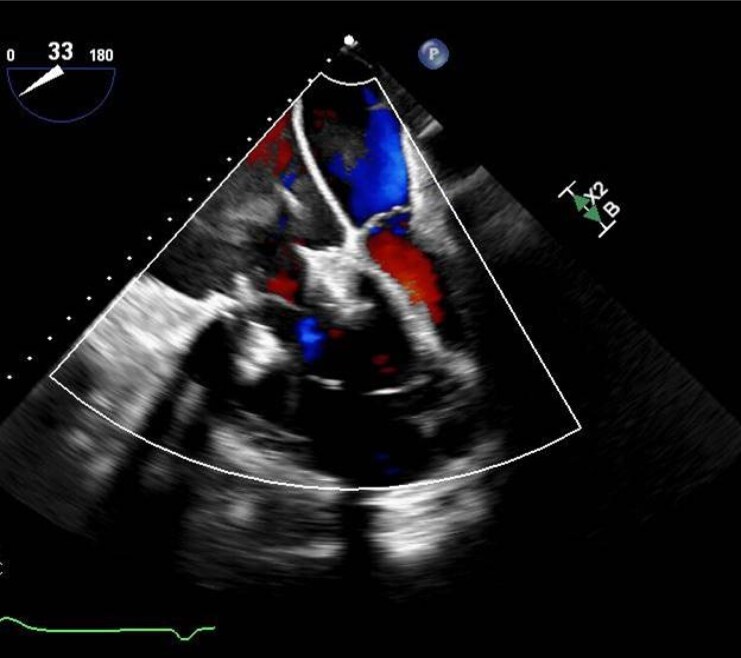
Transoesophageal echocardiography after EVOQUE™ implantation showing no evidence of tricuspid regurgitation.
